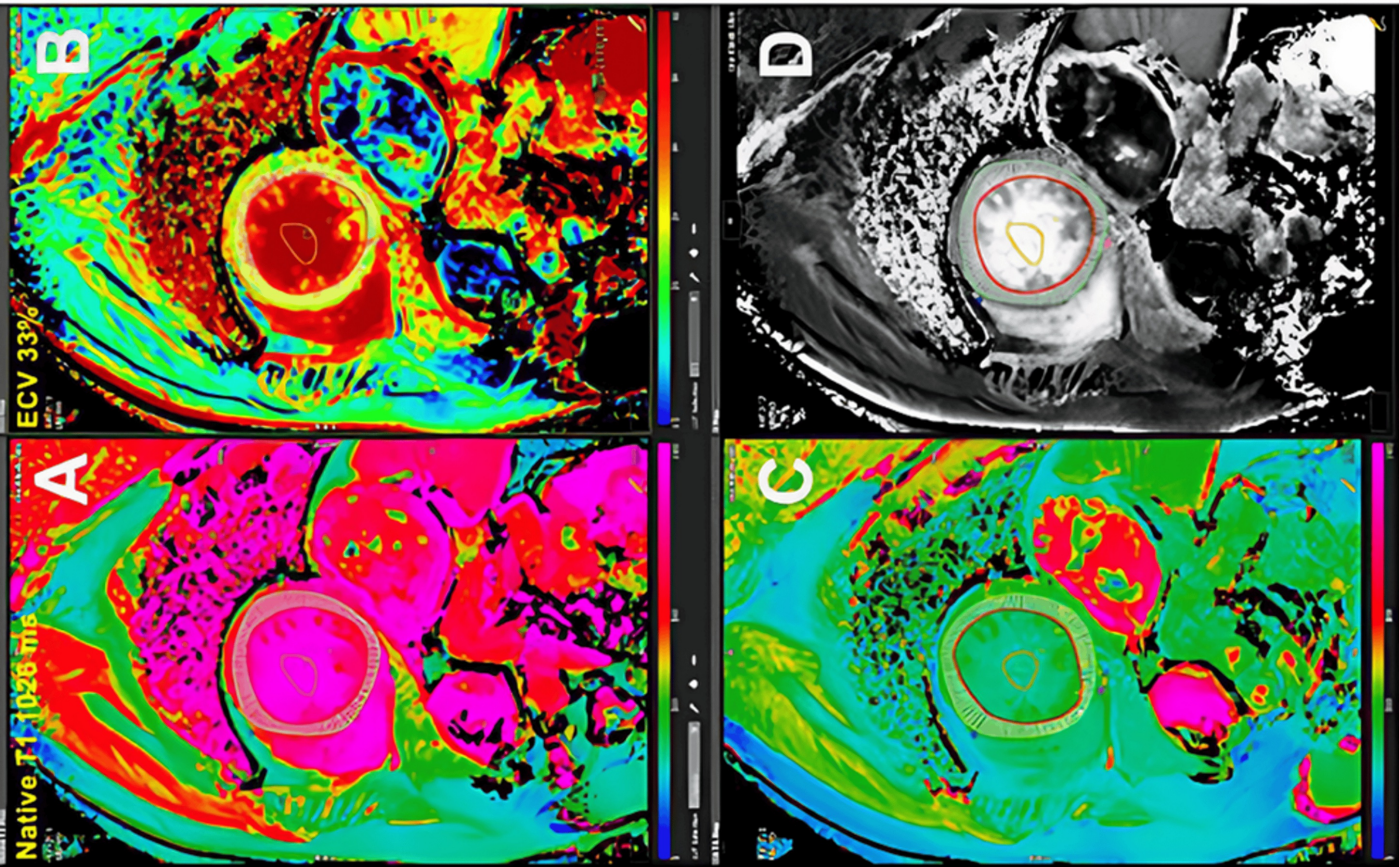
Myocardial T1 mapping and extracellular volume fraction assessment by cardiac magnetic resonance imaging. A) Native T1 mapping, acquired before contrast administration, reflects intrinsic myocardial properties and is useful for identifying diffuse abnormalities such as fibrosis, edema, or infiltrative processes. B) Extracellular volume fraction, a quantitative measure of the myocardial extracellular space derived from pre- and post-contrast T1 values in conjunction with hematocrit, allows assessment of diffuse fibrosis or infiltration. C) Post-contrast T1 mapping, obtained after gadolinium administration, demonstrates contrast distribution within the myocardium and serves as a basis for further quantitative analysis. D) Partition coefficient (λ) map, reflecting the relative distribution of gadolinium between blood and myocardial tissue, is commonly used as a surrogate marker for extracellular volume fraction. Figure reproduced from Gonska et al., licensed under CC BY 4.0 [25].
